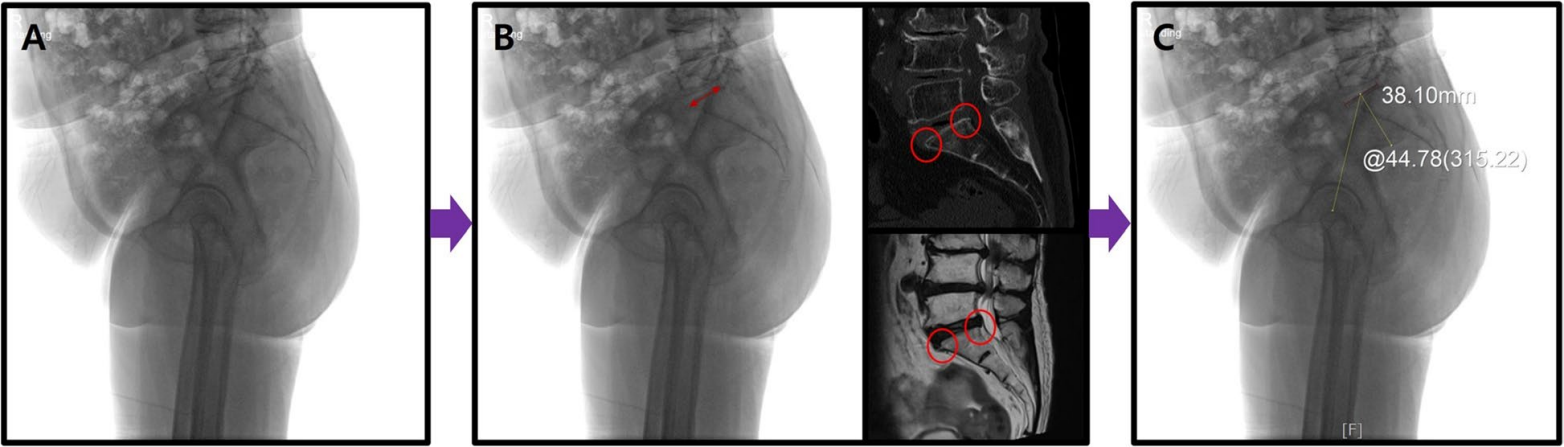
Measurement of pelvic incidence in true pelvis lateral radiographs; A Overlapping of both femoral heads. B Precise identification of sacral endplate using CT or MRI. C More accurate measurement of pelvic incidence
